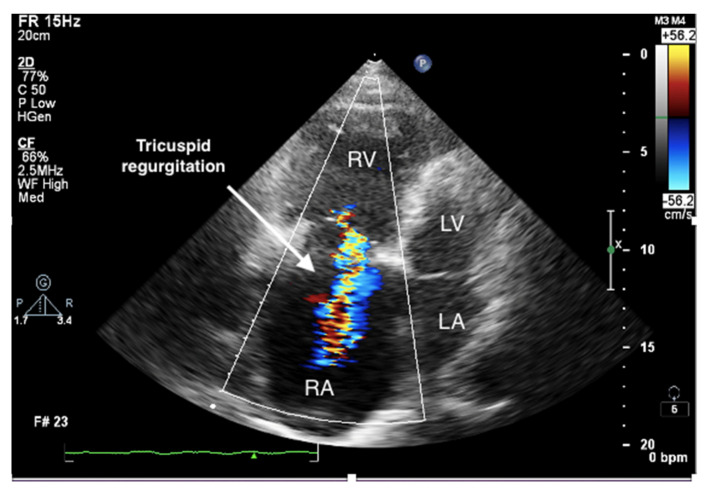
Enlarged right ventricle and right atrium. Tricuspid regurgitation. 2D-TTE, RV-focused apical 4CH view.
